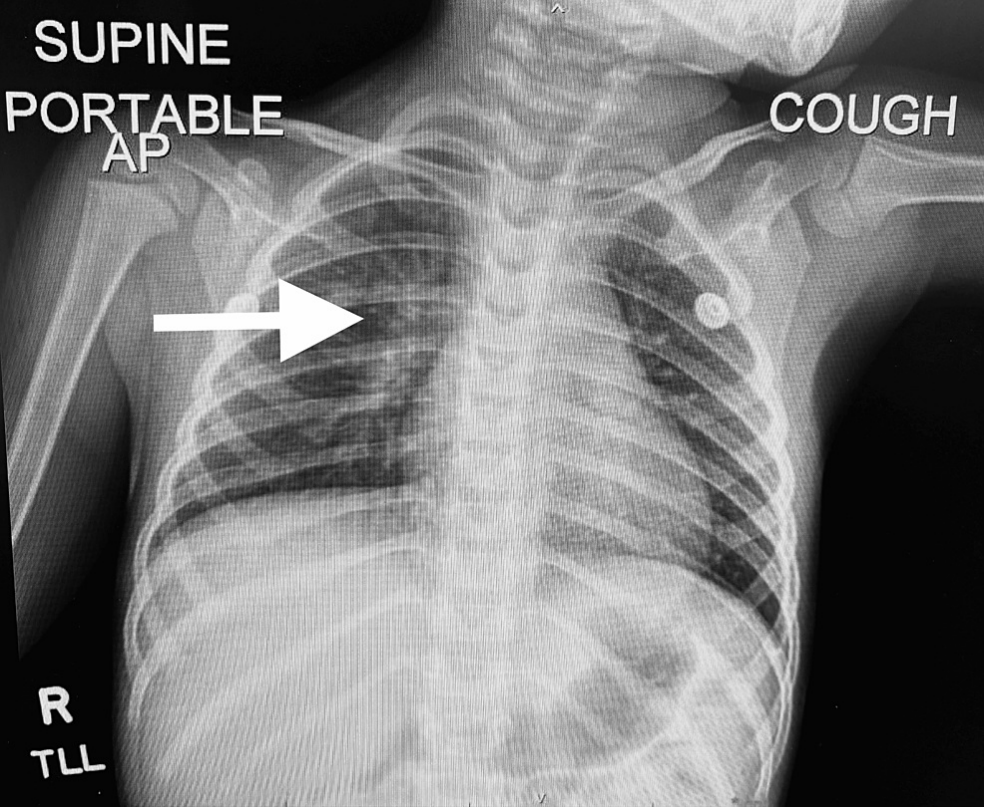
Chest X-ray of the patient at arrival Chest X-ray demonstrates minimal patchy airspace opacity in the right parahilar region
